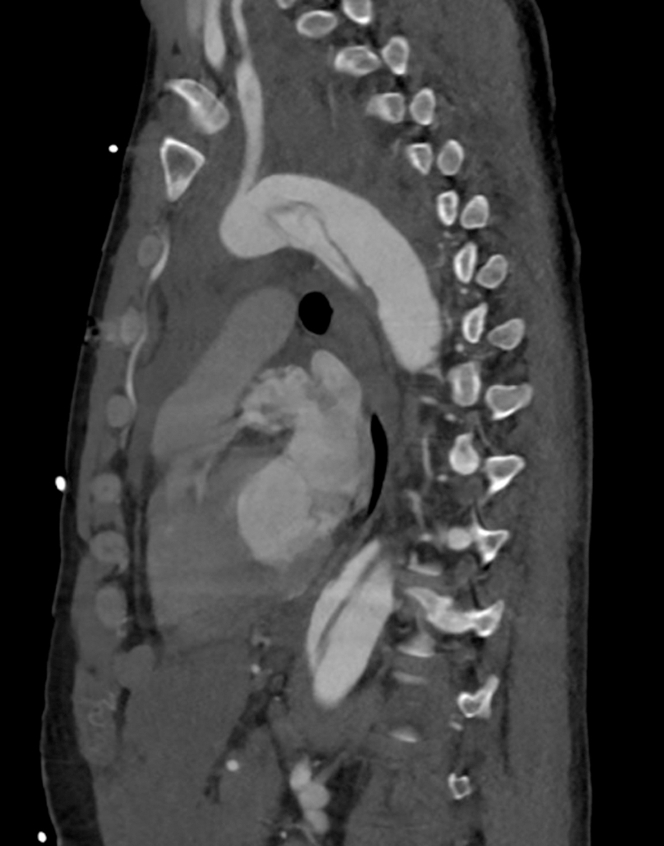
Sagittal Computed Tomography Demonstrating the Extension of Acute Dissection of the Aorta From the Aortic Arch Down to the Descending Aorta
